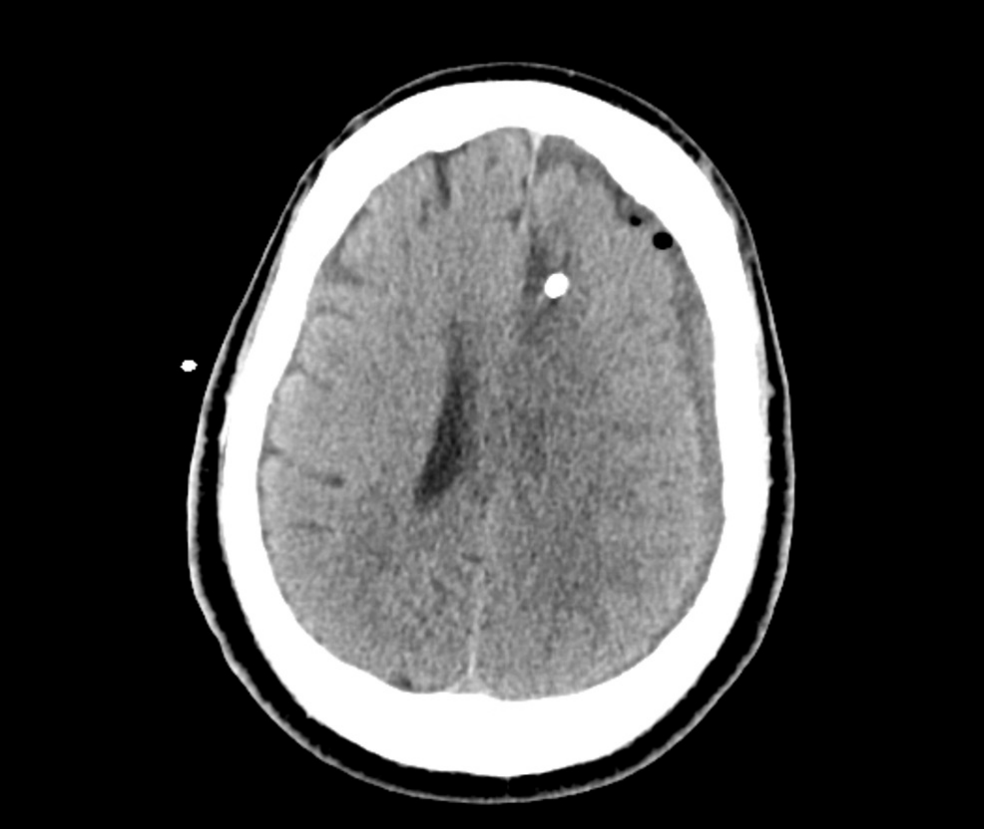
Non-contrast CT demonstrating collapsed abscess cavity and small left frontal extra-axial fluid collection. CT, computed tomography
